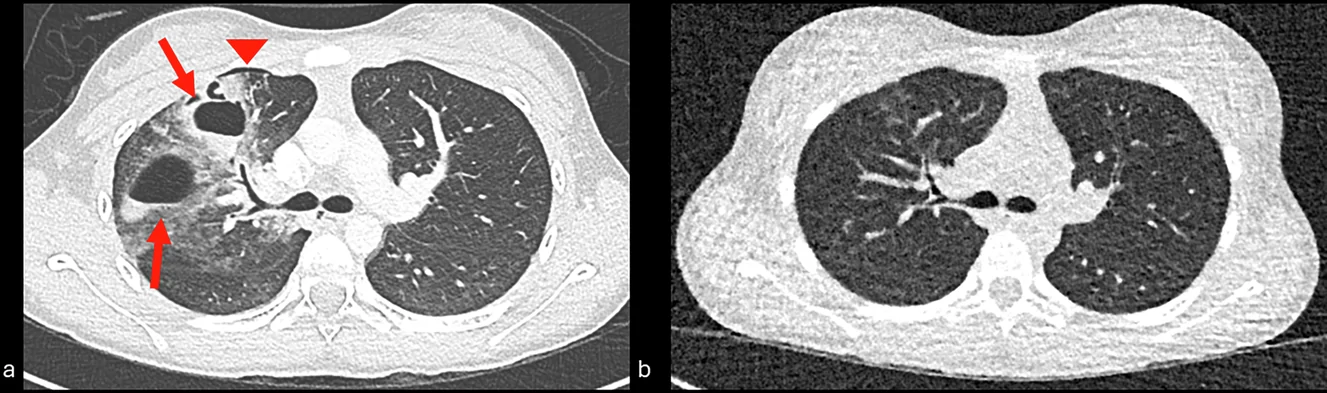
CT scan of a 12-year-old girl with posttraumatic pneumatocele. Normally, a follow-up CT scan is not indicated when asymptomatic. However, the pneumatologist needed to make sure there was no residual air leak because she was going on a diving trip by airplane. Axial (a) image obtained on energy-integrating-detector CT (with intravenous contrast) (CTDI 1.36 mGy, DLP 43 mGycm): pneumatocele (red arrow) and pneumothorax (arrowhead). Axial (b) image obtained on PCCT using ULDCT protocol (without intravenous contrast) when the patient was 13 years old (CTDI 0.01 mGy, DLP 1 mGycm): no residual pneumatocele or pneumothorax
